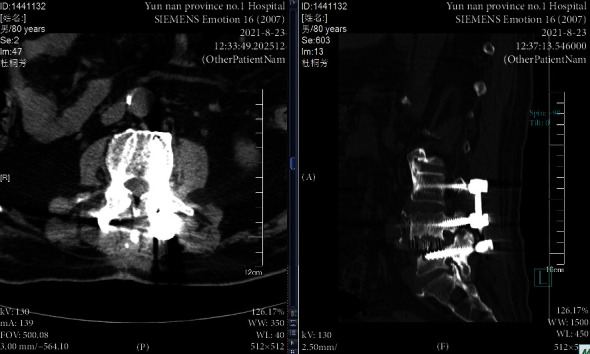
Preoperative CT imaging of the fourth and fifth lumbar spine.
